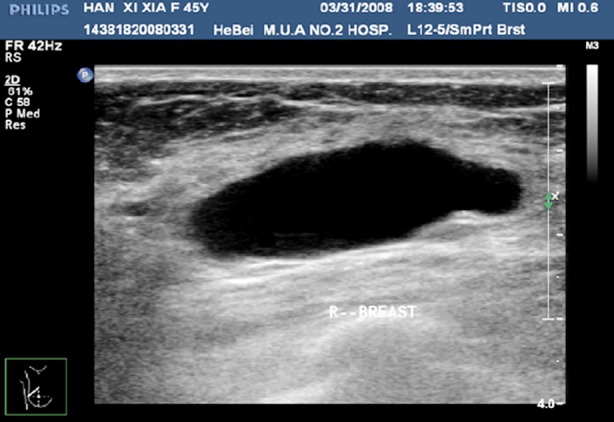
Ultrasonography of benign cyst.
